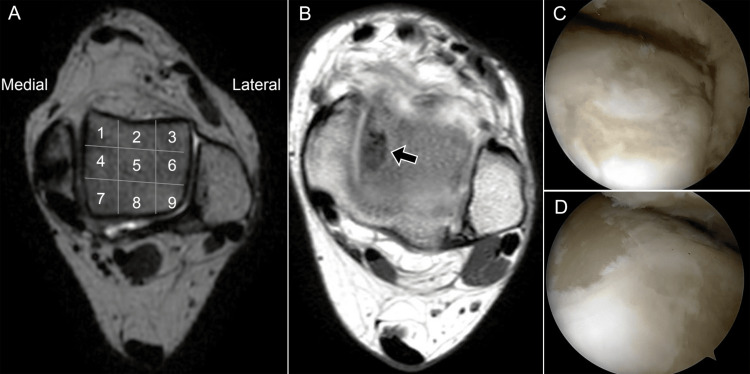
Localization of osteochondral talar lesions. (A) Axial T2 MRI shows the Raikin et al. grid for lesion localization; (B) axial T2 MRI shows chondral denudation in zone 4 (arrow); (C, D) arthroscopy confirms the lesion in the corresponding medial talar dome region. Images belong to the authors; patient consent was obtained.
